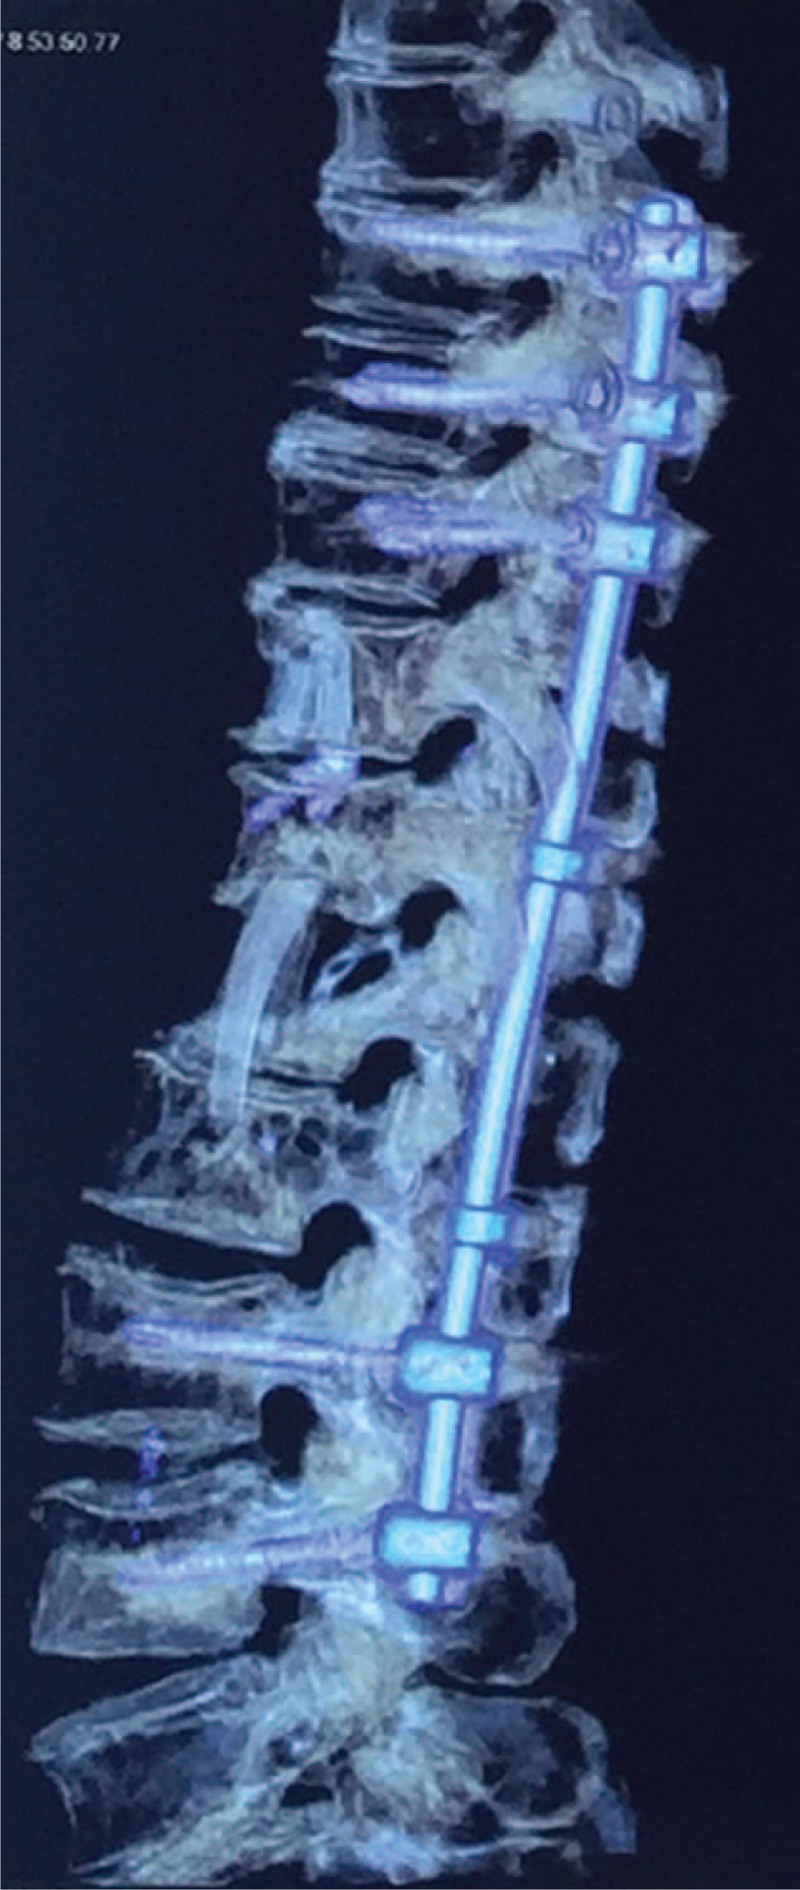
Postoperative 3D CT images after anterior debridement, L1 corpectomy, decompression, interposition of autologous rib graft, and posterior instrumentation of T8–L4. CT = computed tomography.
